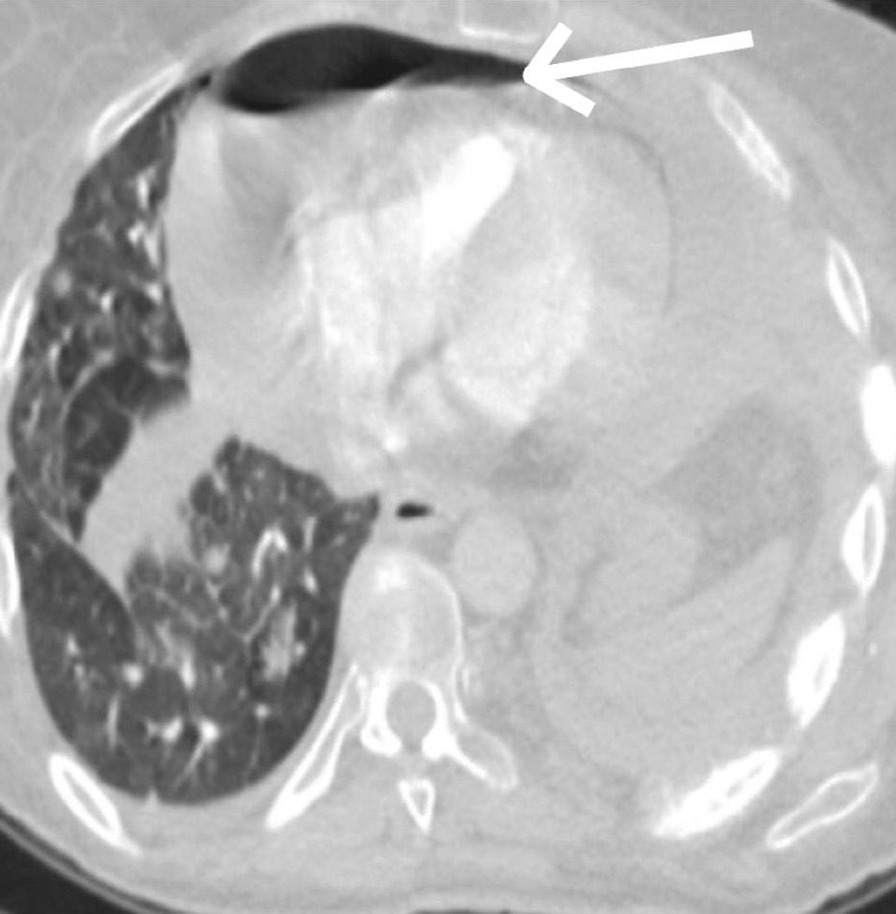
A spiral CT pulmonary angiogram showing heterogeneous soft tissue mass involving left upper and lower lung, with the mass invading the pleural surface and pericardium. The arrow refer to heterogeneous soft tissue mass involving left upper and lower lung
